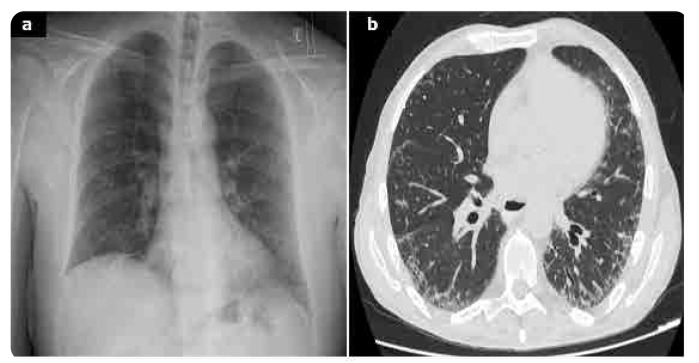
A 65year-old man, ex-smoker with seropositive RA with dry cough and dyspnoea on exertion. Antero-posterior x-rays of the chest reveals mild reticular opacities mainly in the lower zones with small amount of pleural effusions on the right side (a). Axial CT scan of the chest with lung window settings shows mild peripheral reticular opacities and traction bronchiectasis, without honeycombing compatible with a UIP pattern (b).
